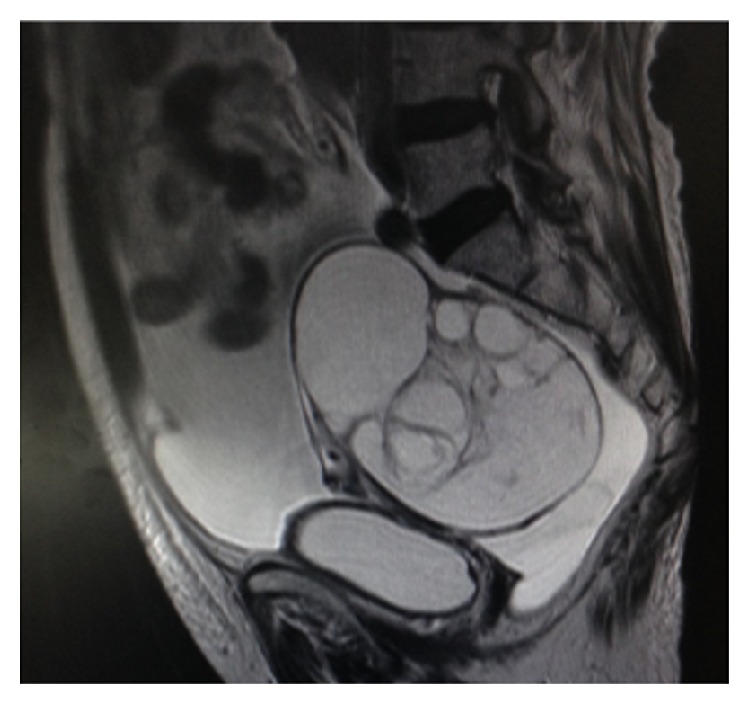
MRI image of patient 3 before operation.
